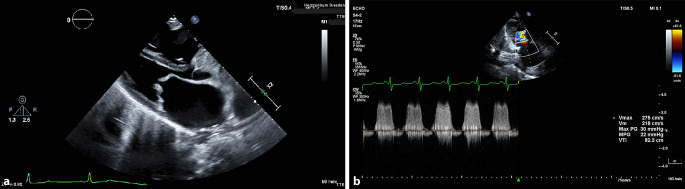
a Parasternal long-axis view of the mitral valve stenosis with transthoracic echocardiography. b Pressure measurement of the mitral valve stenosis with continuous wave (CW) doppler with transthoracic echocardiography
